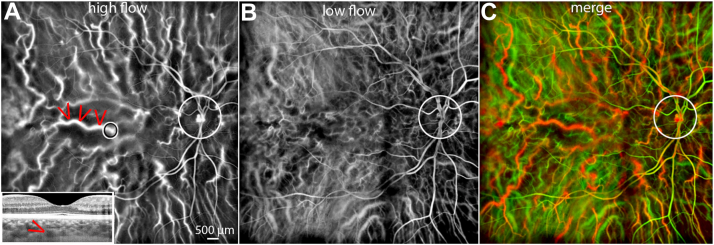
Representative large field laser Doppler holography imaging of a 33-year-old man (refraction –3 diopter; choroidal thickness 240 μm). The field of view is 40°. A, B, High- and low-velocity maps showing, respectively, arteries (Doppler shift 26–39 kHz) and small arterioles and veins (2–10 kHz). The small circle indicates the fovea, the large circle the optic nerve, and the red arrowheads the submacular artery (SMA). The insert in A is a vertical OCT scan showing the SMA surrounded by an avascular halo. In C, the red-green merged image demonstrates minimal overlap between arteries and veins.
